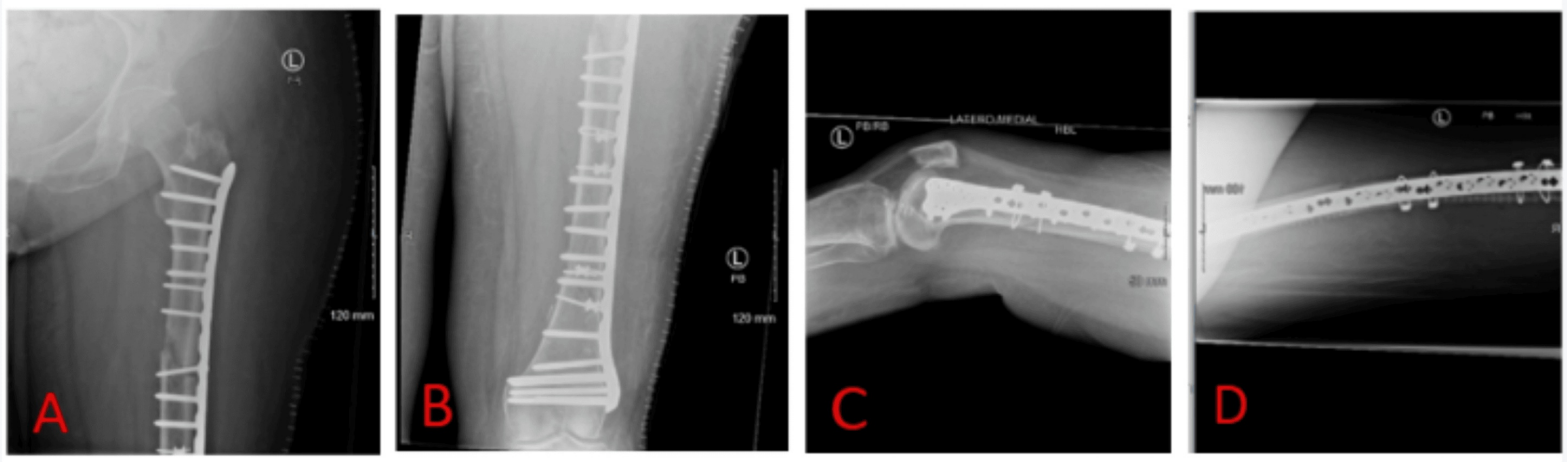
Postoperative X-rays after the first revision surgery (ORIF) from the secondary treating surgeon A: Anteroposterior X-ray of the left proximal femur; B: Anteroposterior X-ray of the left distal femur; C: Lateral X-ray of the left distal femur; D: Lateral X-ray of the left proximal femur ORIF: open reduction and internal fixation
